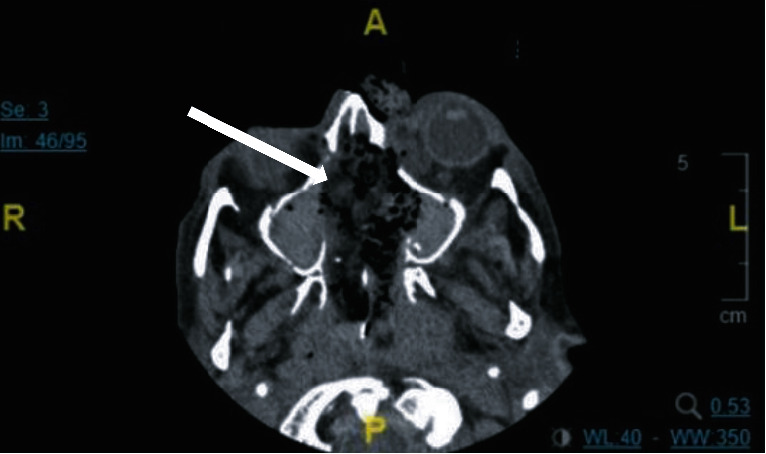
Patient 3 MRI image mucromycosis growth in sinuses.
